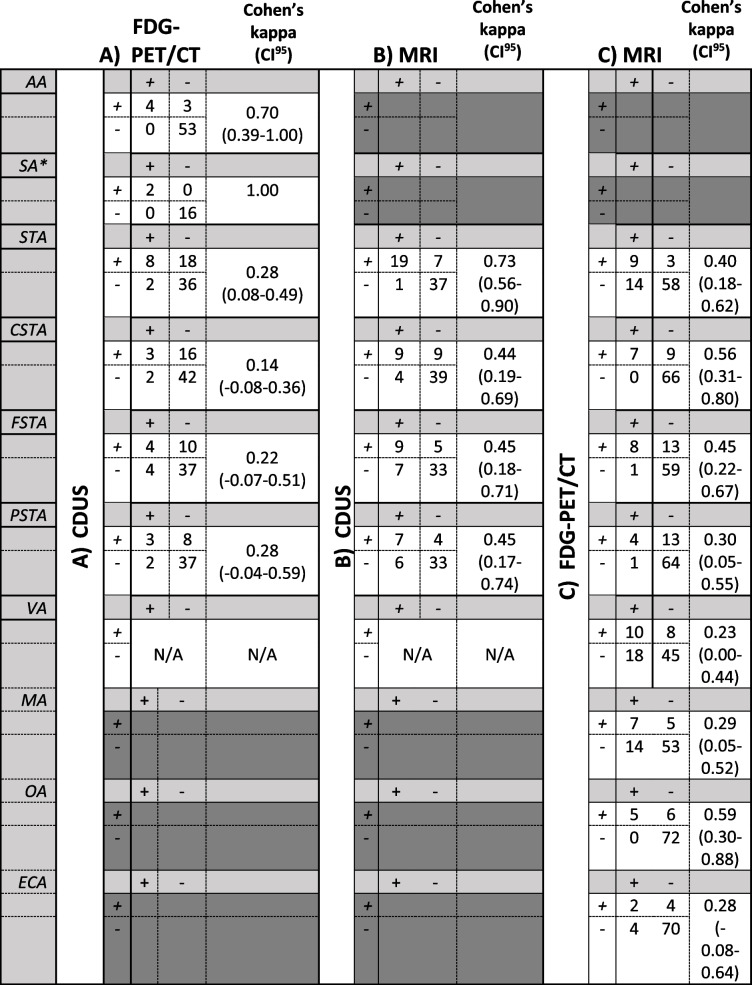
Cross tabulation of the CDUS, FDG-PET/CT and MRI assessments for bilateral arteries (reliably) assessable for at least 2 of the imaging modalities, using the consensus score of the two experts. Total numbers vary as not all arteries were assessable in every patient on multiple modalities. Comparisons with CDUS was performed in 32 patients. Cohen’s kappa and its 95%-confidence interval (CI95) are shown in the right column. A) CDUS comparison with FDG-PET/CT for the axillary artery and the branches of the superficial temporal artery. No patients were CDUS and FDG-PET/CT negative in the vertebral arteries, which precluded calculation of kappa statistic. B) CDUS comparison with MRI for the branches of the superficial temporal and the vertebral artery. No patients were CDUS and MRI negative in the vertebral arteries, which precluded calculation of kappa statistic. C) FDG-PET/CT comparison with MRI for the branches of the superficial temporal, the maxillary, occipital and external carotid artery
